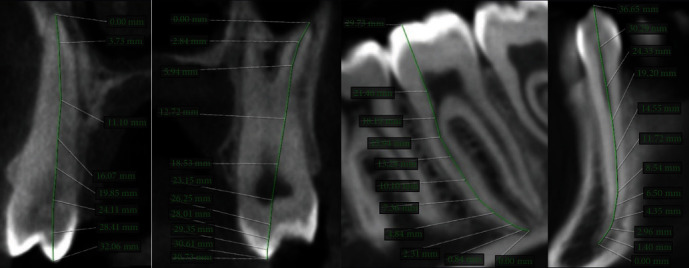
Sagittal section from cone beam computed tomography (CBCT) showing tooth sizes with radiculomegaly.
